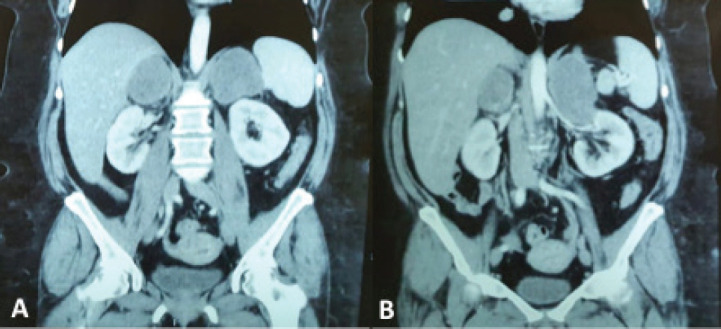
(a and b) Coronal sections on computed tomography (CT) scans showing bilateral adrenal masses (right of 66 mm and left of 106 mm).
